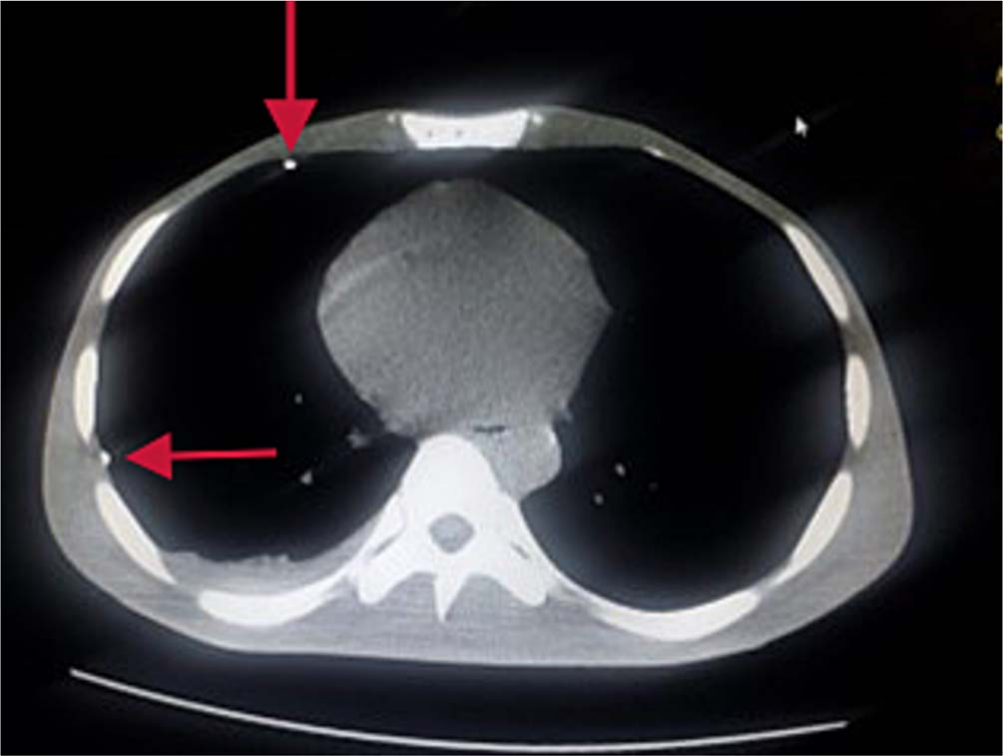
Thorax CT showing guide wire in the pleural space.
